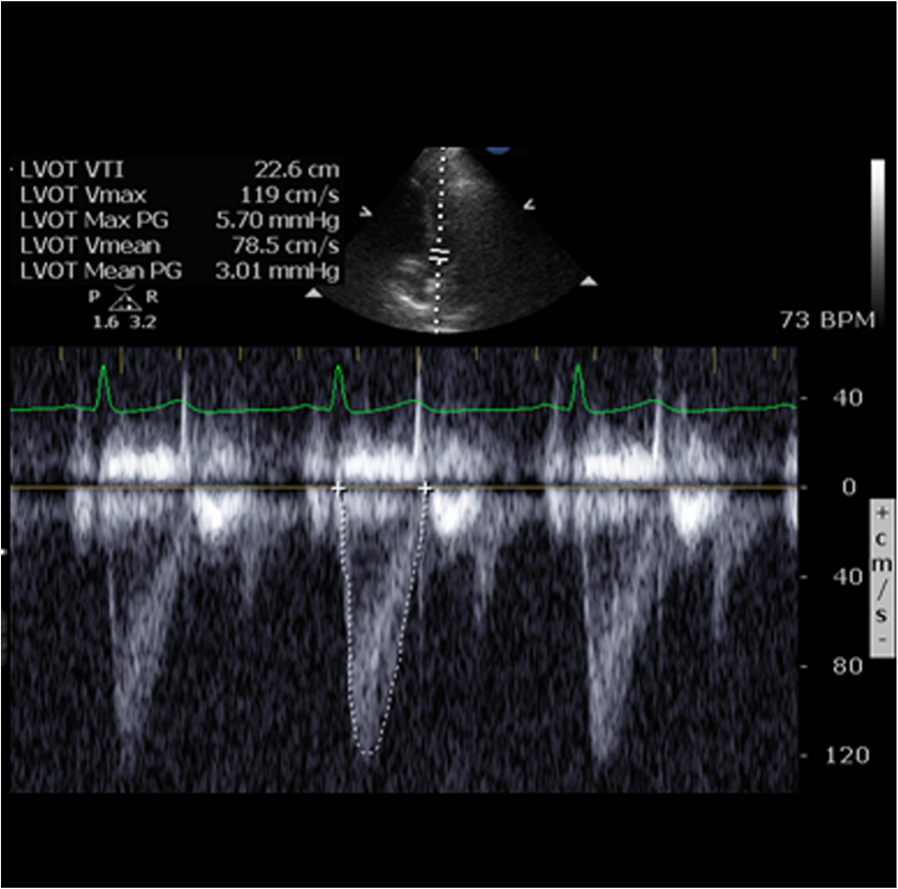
Example image of pulse wave Doppler in the LVOT and measurement of VTI tracing
